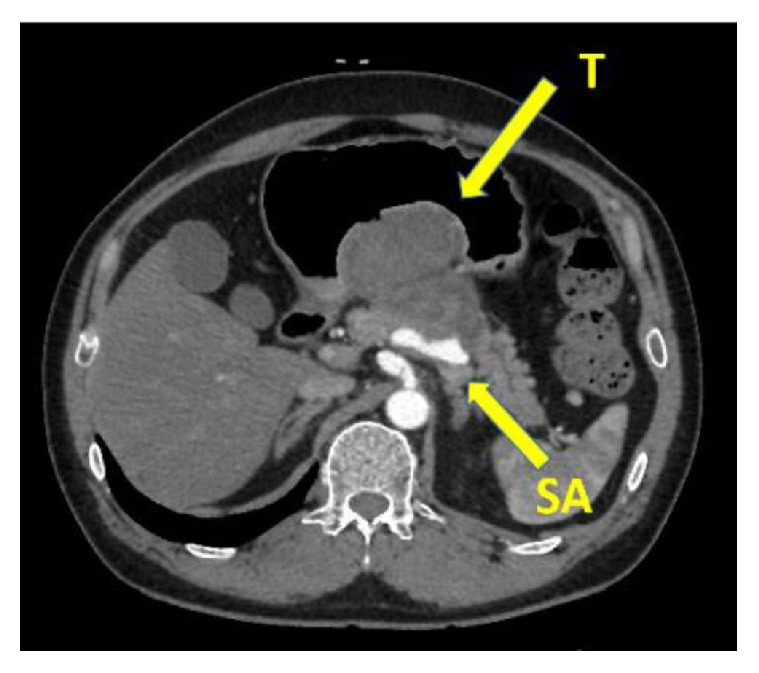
Computed tomography indicated a tumor measuring 65 × 50 mm in the stomach, which was suspected to have invaded into the pancreas, but no metastatic lesions were detected. T: tumor; SA: splenic.
